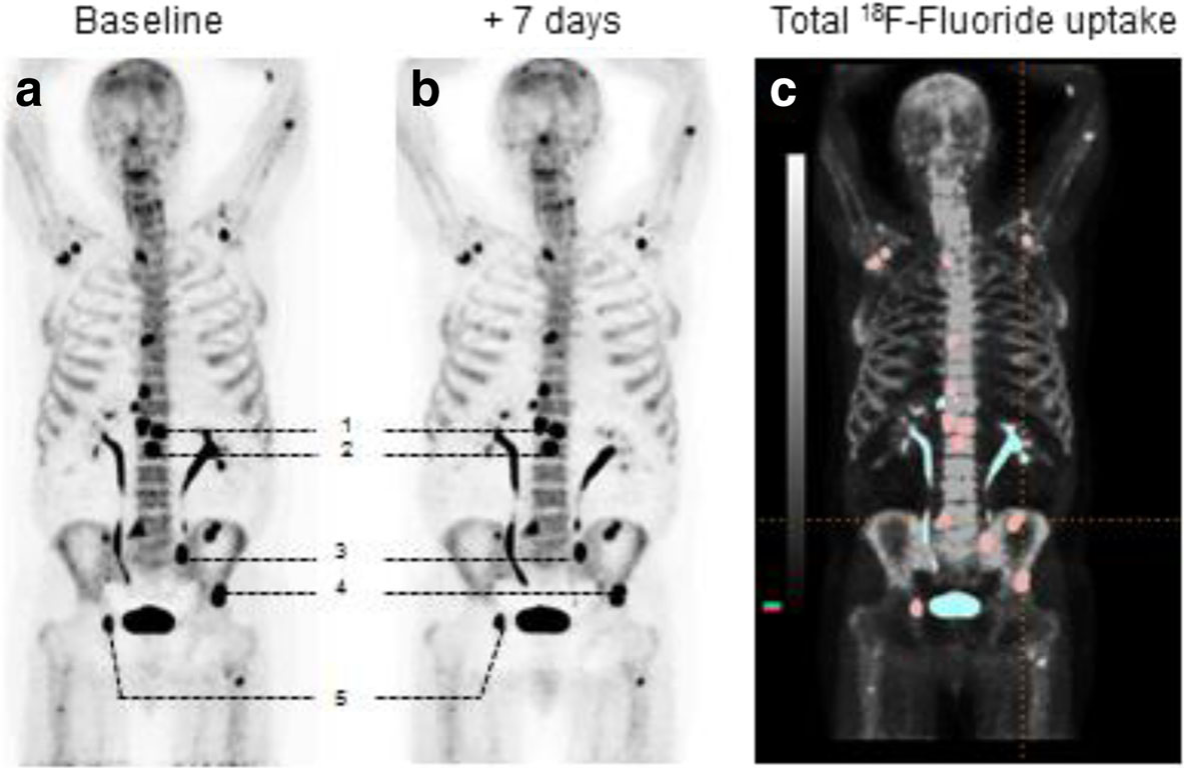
Patient 8 at baseline (a) and at +7 days (b) performing PET1 and PET2. Five chosen index lesions (numbered 1–5) were delineated for VOI measuring SUVmax, SUVmean, FTV_50%_ and total lesion fluoride uptake at PET/CT (baseline). c Included lesions are highlighted in *red* (*n* = 17) and the *blue* uptake represents lesions that were omitted, urine activity and degenerative or equivocal findings
